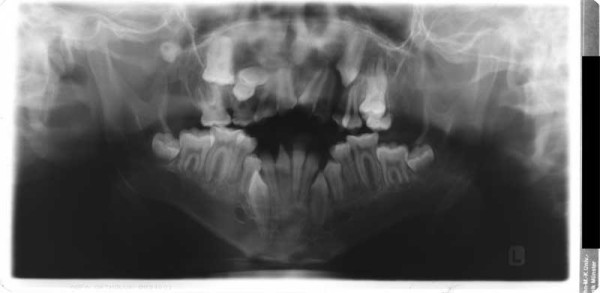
Age 13.2. Panoramic x-ray. Congenitally missing tooth 28 and impacted teeth 18, 17, 14, 13, 23, 27, 38, 33, 43 and 48. Because of extreme crowding, impaction, and space discrepancy, extraction of teeth 17, 14, 13, 23, 38, 33, 43, and 48 were considered. Tooth 18 will be left in place because of its malposition.
